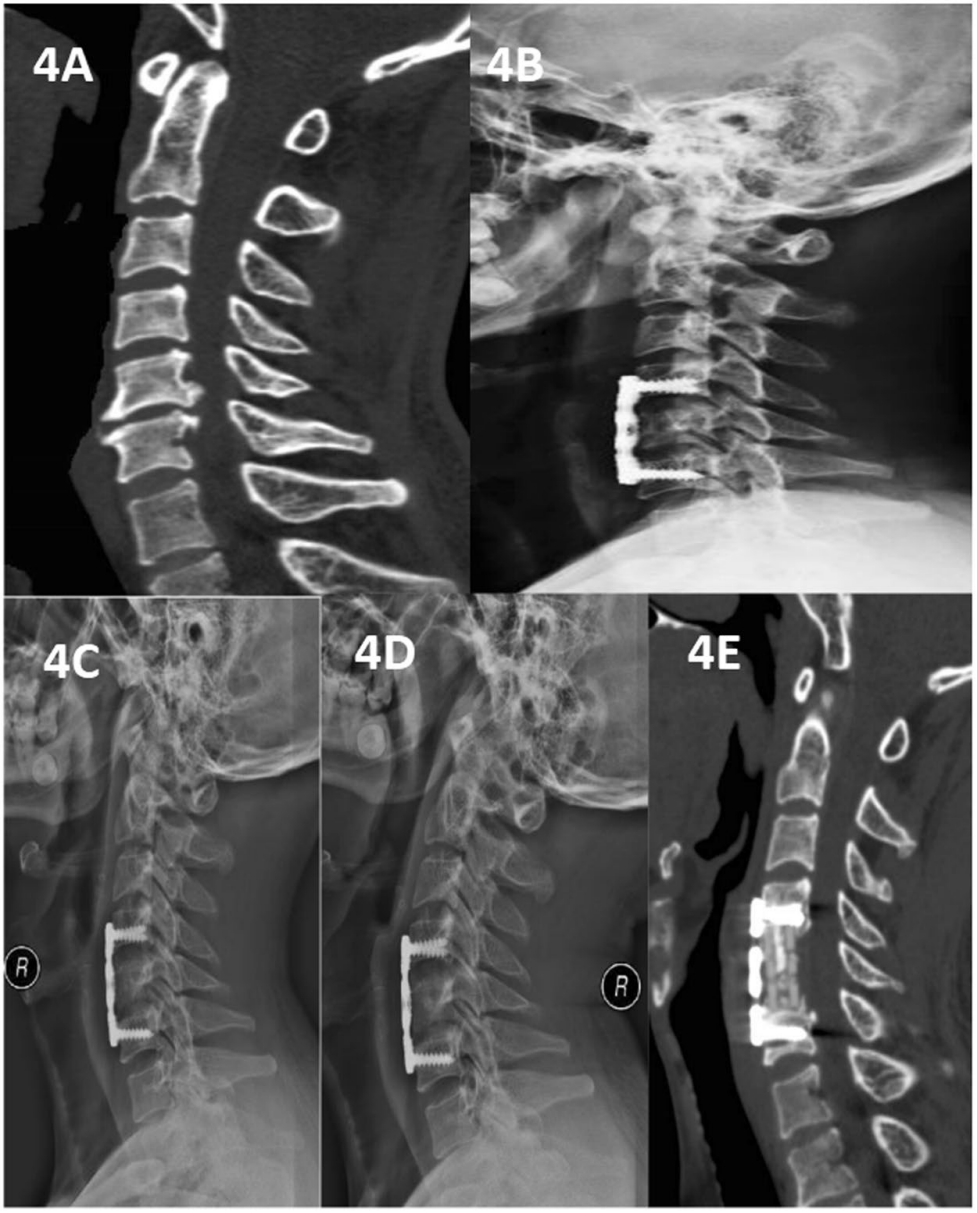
A 55-year-old man who underwent 1-level corpectomy with a nanohydroxyapatite/polyamide66 strut for cervical reconstruction. The preoperative cervical CT (A) revealed C5/6 disc herniation and osteophyte proliferation. The patient underwent a C5 corpectomy and fusion using an n-HA/PA66 strut (B). The lateral X-ray film and CT indicated that the autogenous bone granules filling the strut had achieved bony fusion, and subsidence was observed at the 6-month follow-up (C,E). The strut and internal fixation were in position at 3, 6, and 12 months of follow-up (B–D).
